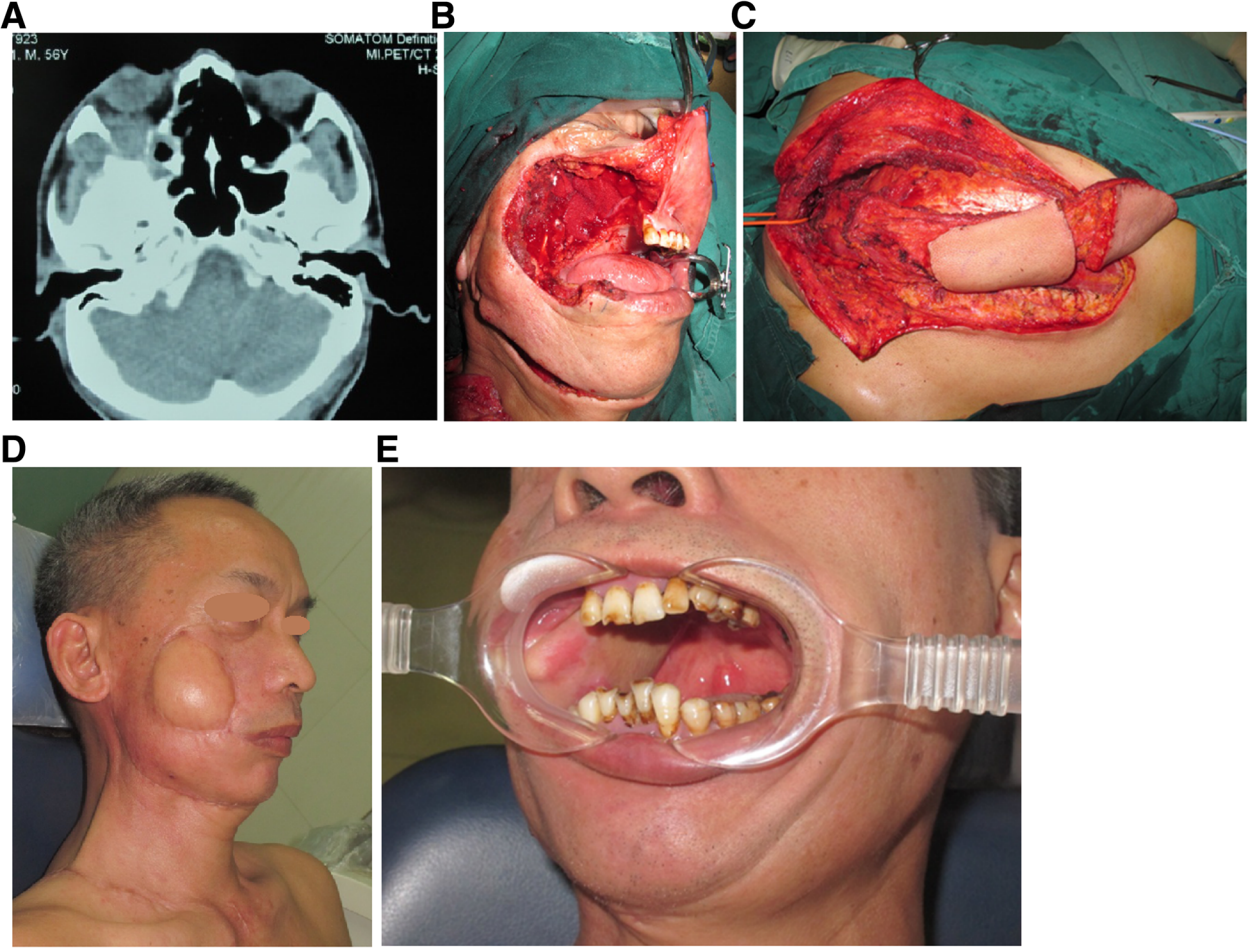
A 56-year-old man with recurrent palatal ACC underwent salvage surgery, including en bloc resection and reconstruction with an extended lower vertical trapezius island myocutaneous flap (TIMF). Computed tomography scan showing that the tumor invaded the middle cranial fossa and posterior cranial fossa (A). The patient underwent en bloc resection (B), and the extended lower vertical TIMF was harvested (C). The folded flap provided an inner and outer lining for repair of the through-and-through defect of the major craniomaxillofacial region (D, E)
